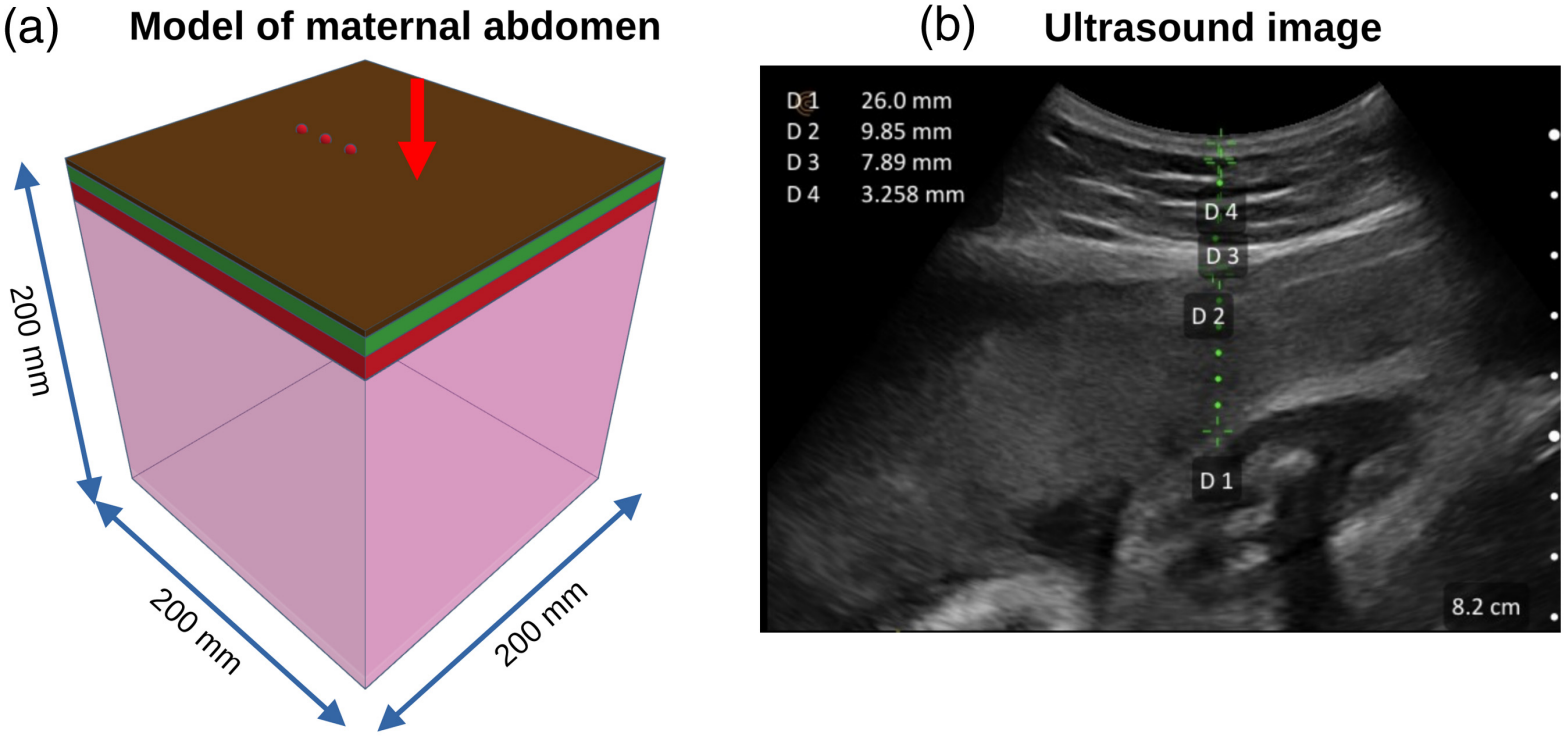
Developing a model of maternal abdominal structures. (a) Model of a maternal abdomen and acquisition setup using the adjustable distance broadband NIRS device Mini CYRIL.^26^ The dark green, light green, purple, and blue layers represent the skin, adipose tissue, muscle, and placenta layers, respectively. The light source is depicted as a red arrow, and the three different positions of the fiber detector of the adjustable-distance CW-NIRS device are shown as red disks (source–detector separations of 30, 40, and 50 mm). (b) Ultrasound image used for measuring layer thickness (D1: placenta thickness, D2: muscle thickness, D3: adipose tissue thickness, D4: skin thickness). In the rest of the paper, the term placenta depth refers to the distance from skin to placenta: D2+D3+D4
